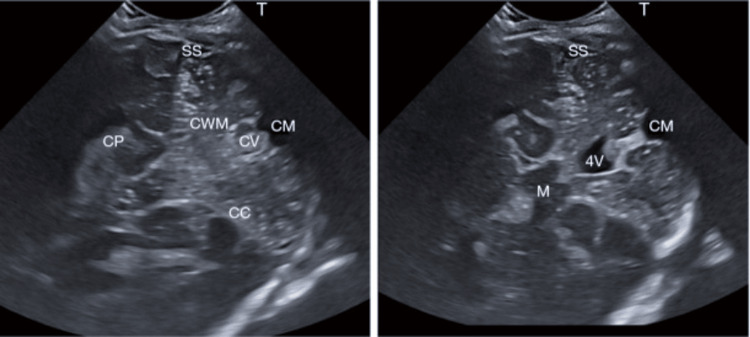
Coronal ultrasonographic images of the posterior fossa were obtained through the mastoid fontanelle in the same patient. The images show the cerebellar hemispheres, including cortical and white matter, cerebellar vermis, sigmoid sinus, fourth ventricle, and cisterna magna. Above the tentorium, the temporal lobe and temporal horn of the lateral ventricle are visible, with the choroid plexus identified within the ventricular cavity. Reproduced from Caro-Domínguez P et al.: distributed under the terms of the Creative Commons Attribution-NonCommercial-NoDerivatives 4.0 International License (CC BY-NC-ND 4.0).
